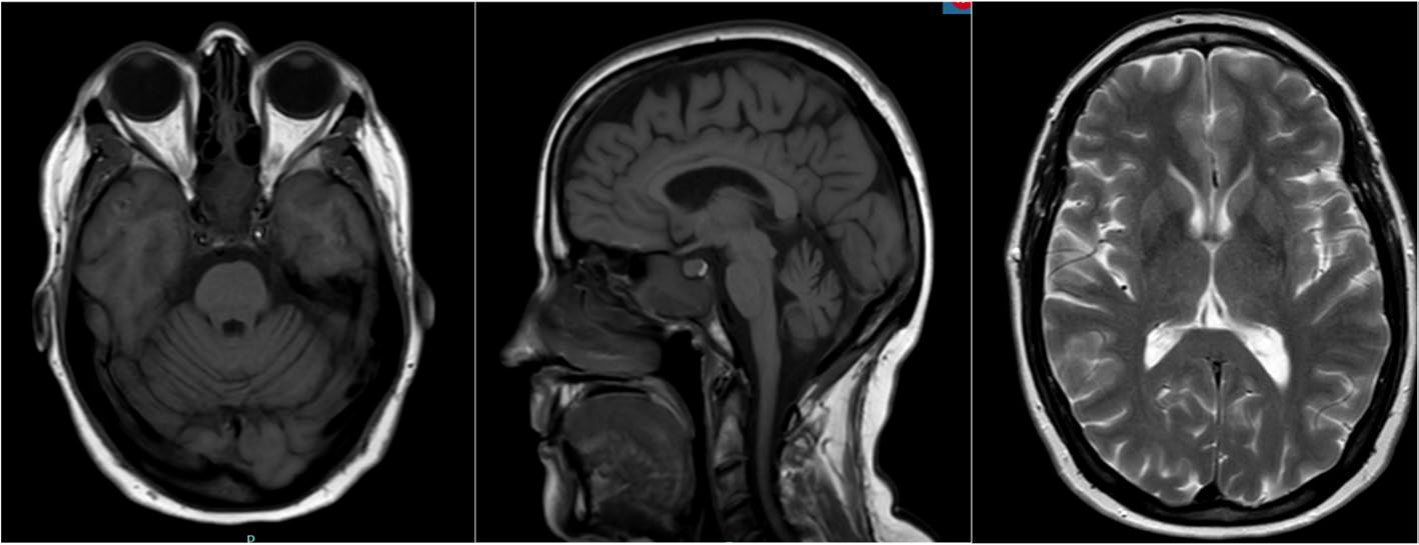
MRI brain imaging nine months after symptomatic onset. Left to right–T1 axial view; T1 sagittal view; T2 axial view
